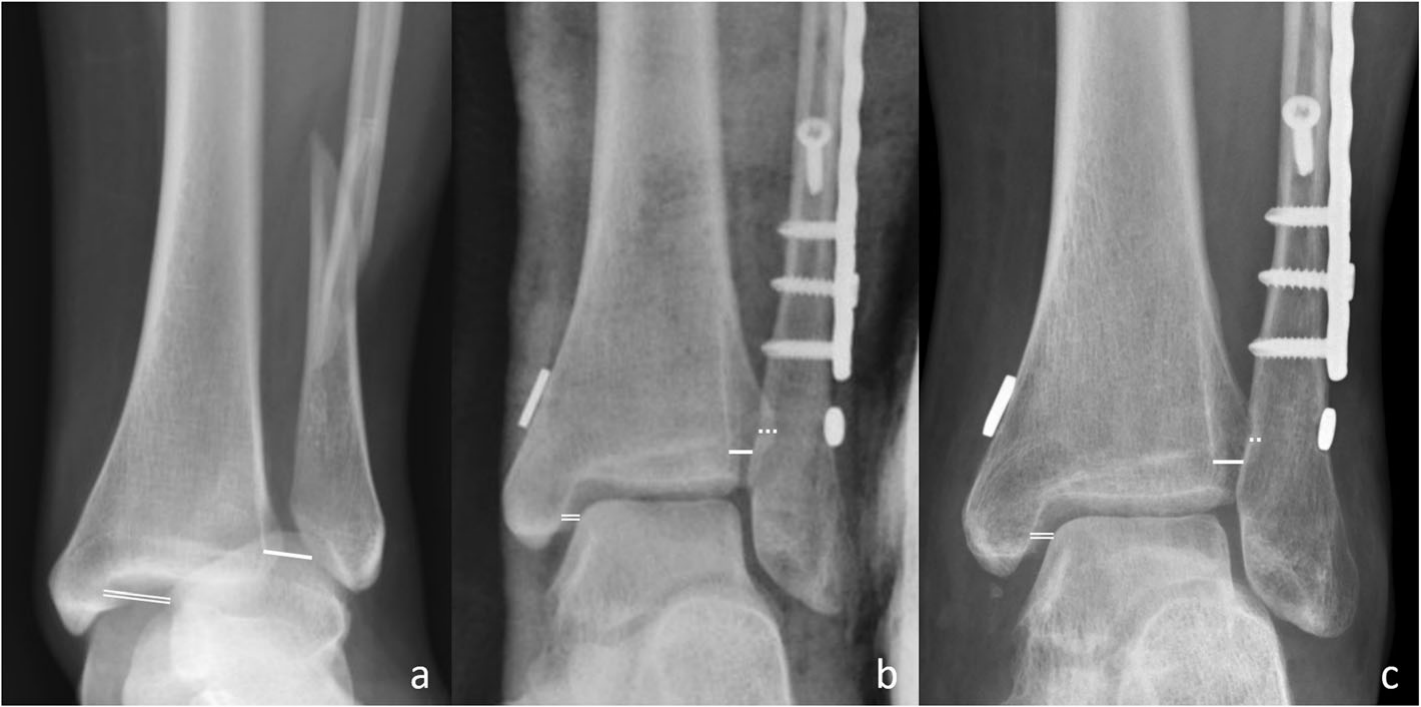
Exemplary X-ray images of the measurements commenced at different time points, preoperative (a), postoperative (b), final follow-up (c). The firm lines represent measurements of the tibiofibular clear spaces (TFCS), the double firm lines represent measurements of the medial clear spaces (MCS), and the dotted lines represent measurements of the tibiofibular overlaps (TFO)
